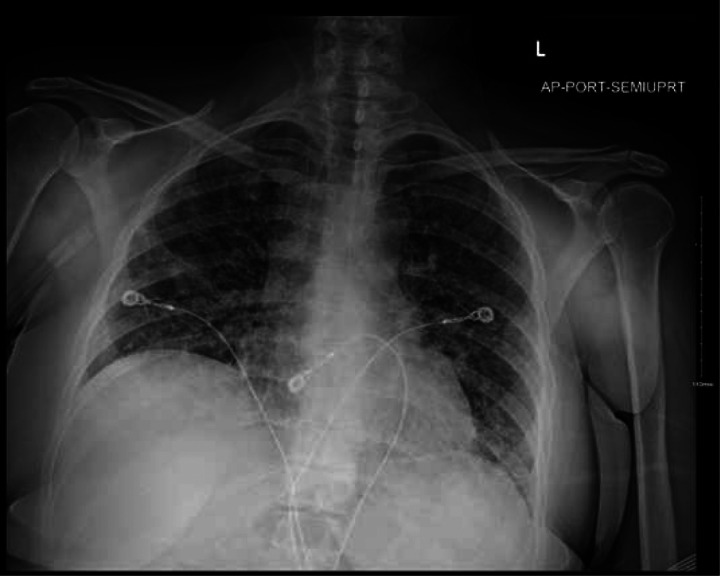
Portable chest X-ray on admission the patient’s second admission to the hospital.
